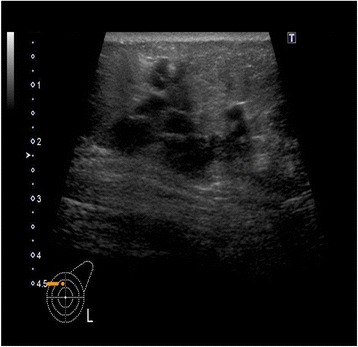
Ultrasonography (US). A US image of the breast shows an ill-defined, irregular, and hypervascular hypoechoic tumor measuring 36 × 22 × 22 mm
